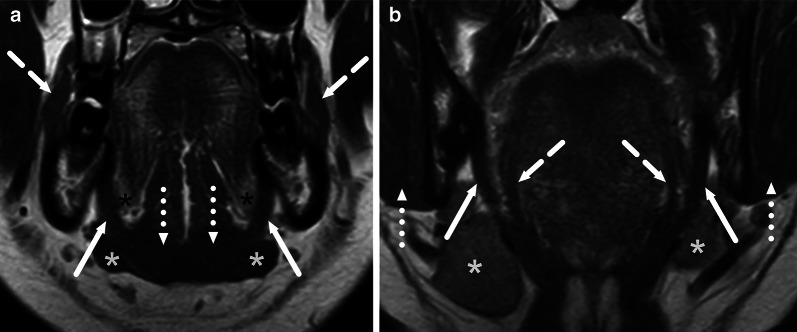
Normal MRI anatomy of the oral cavity. a A coronal T2-weighted image at the anterior part shows the mylohyoid muscle (arrow), separating the sublingual space superiorly including the sublingual gland (black asterisk) and the submandibular space inferiorly. Medially, and superior to the mylohyoid muscle, are the geniohyoid muscles (dotted arrow) and genioglossus muscles (superior to the geniohyoid muscles). Inferiorly, images show the anterior belly of the digastric muscle (white asterisk), and laterally, the buccinator muscle (dashed arrow). b A coronal T2-weighted image at a more posterior part shows the mylohyoid muscles (arrow) and the hyoglossus muscles more medially (dashed arrow), and the masseter muscle laterally in the masticator space (dotted arrow). Submandibular glands (asterisk) are shown below the mylohyoid muscle
